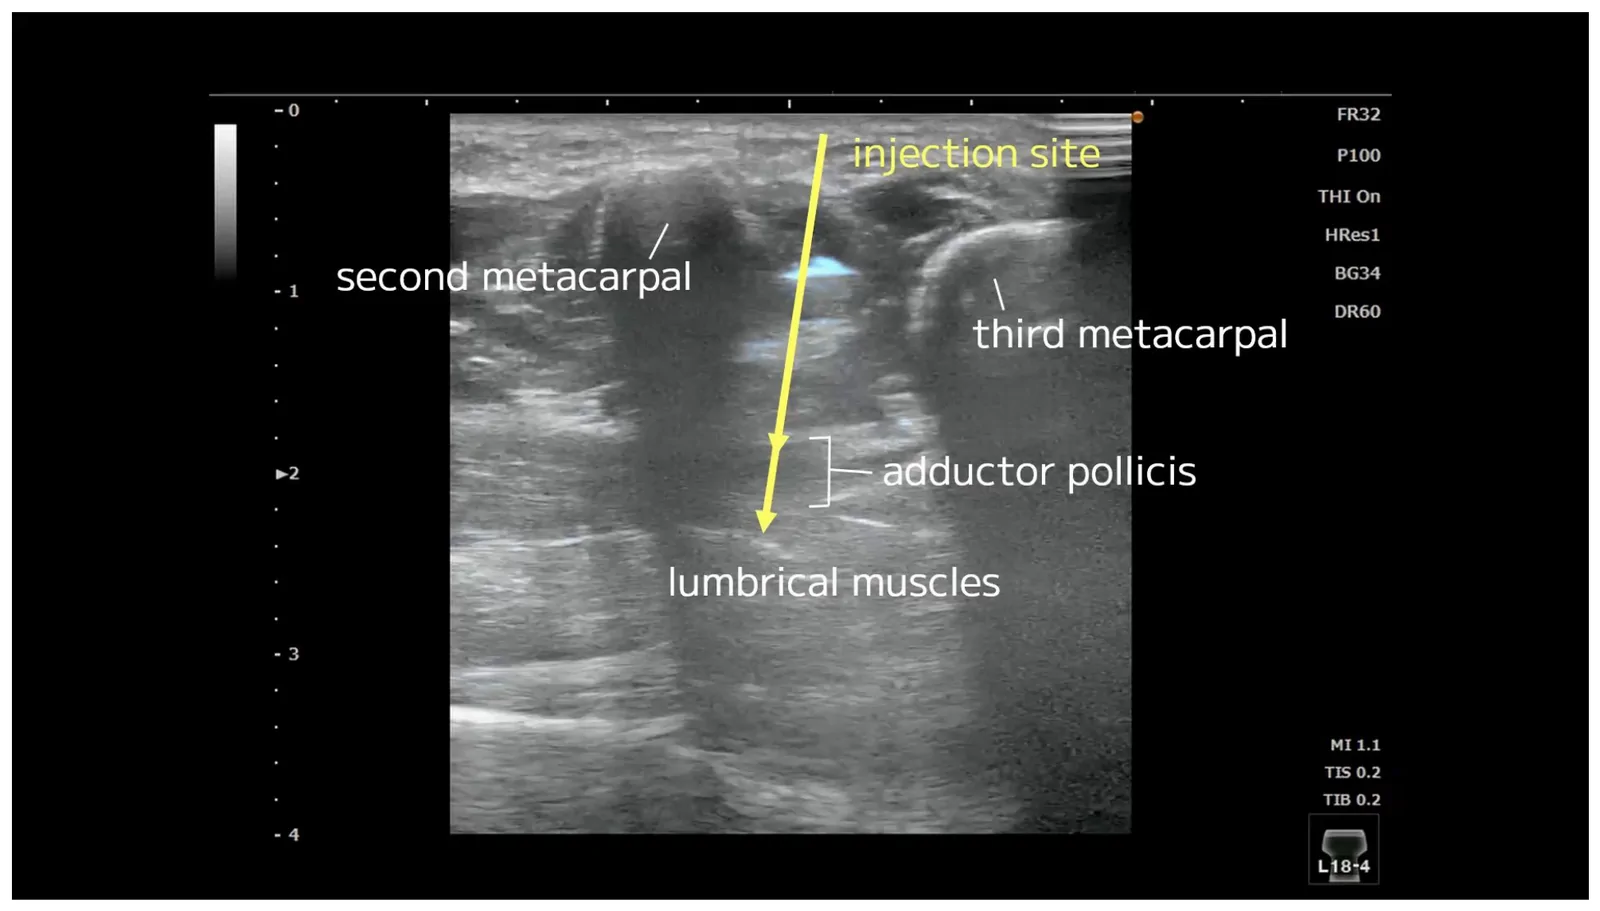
Point 8—adductor pollicis (oblique + transverse heads): ultrasound-guided fascia hydrorelease; yellow arrow indicates the injection site.
